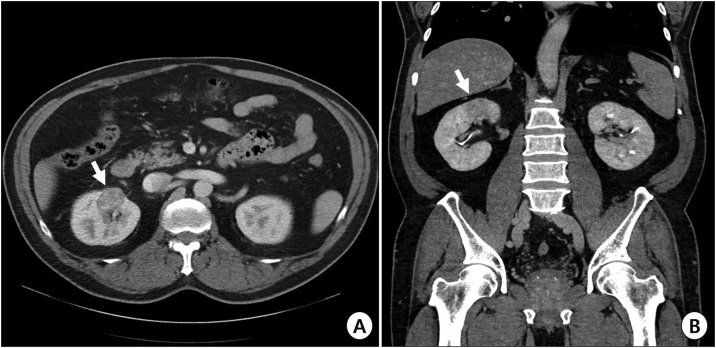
Preoperative kidney dynamic computed tomography scan. a) Axial view. b) Coronal view. Arrows indicate the mass lesion located in the right kidney upper pole mimicking renal cell carcinoma.
